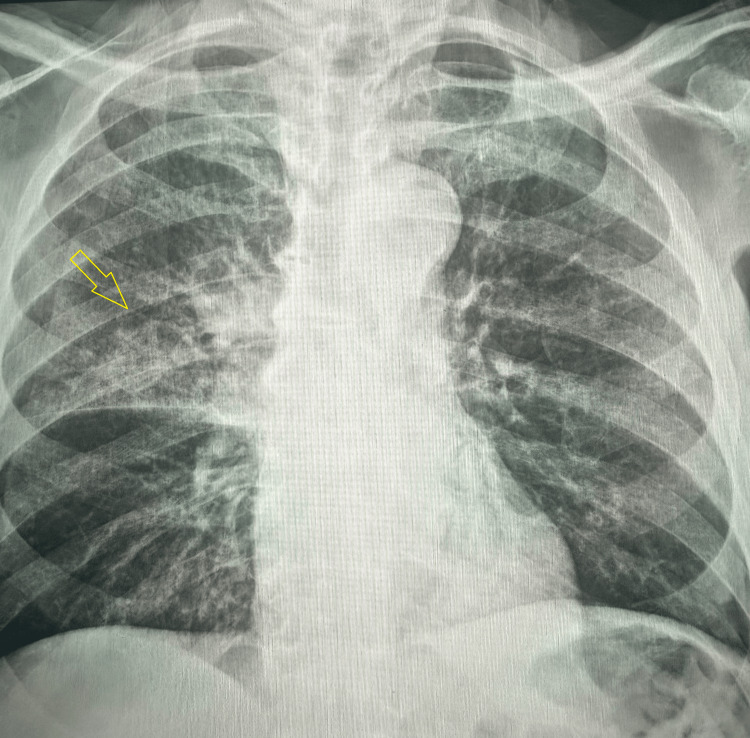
Chest X-ray findings. Chest radiograph demonstrating a right perihilar infiltrates (yellow arrow) consistent with pneumonia.
